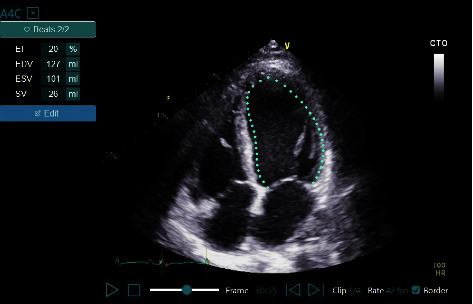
LVivo EF: AI-based tool for automated LVEF assessment from apical 4-chamber view echocardiographic clips. AI, artificial intelligence; LVEF, left ventricular ejection fraction.
